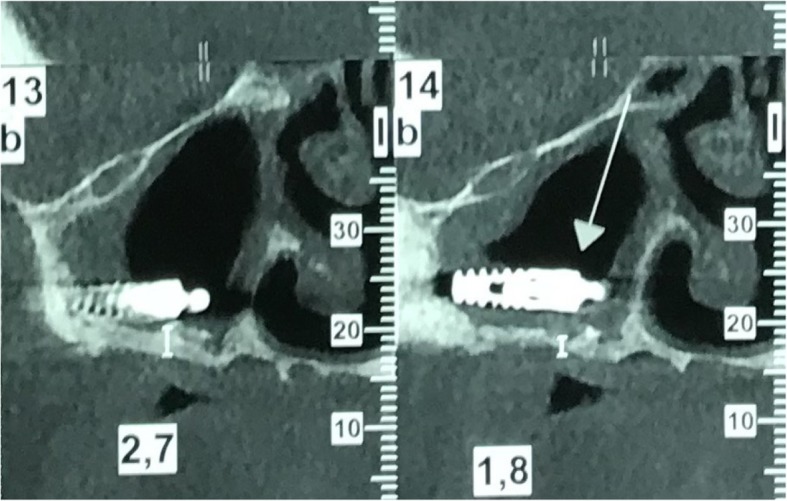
The exact position of the displaced implant into the maxillary sinus was made known after performing CBCT
